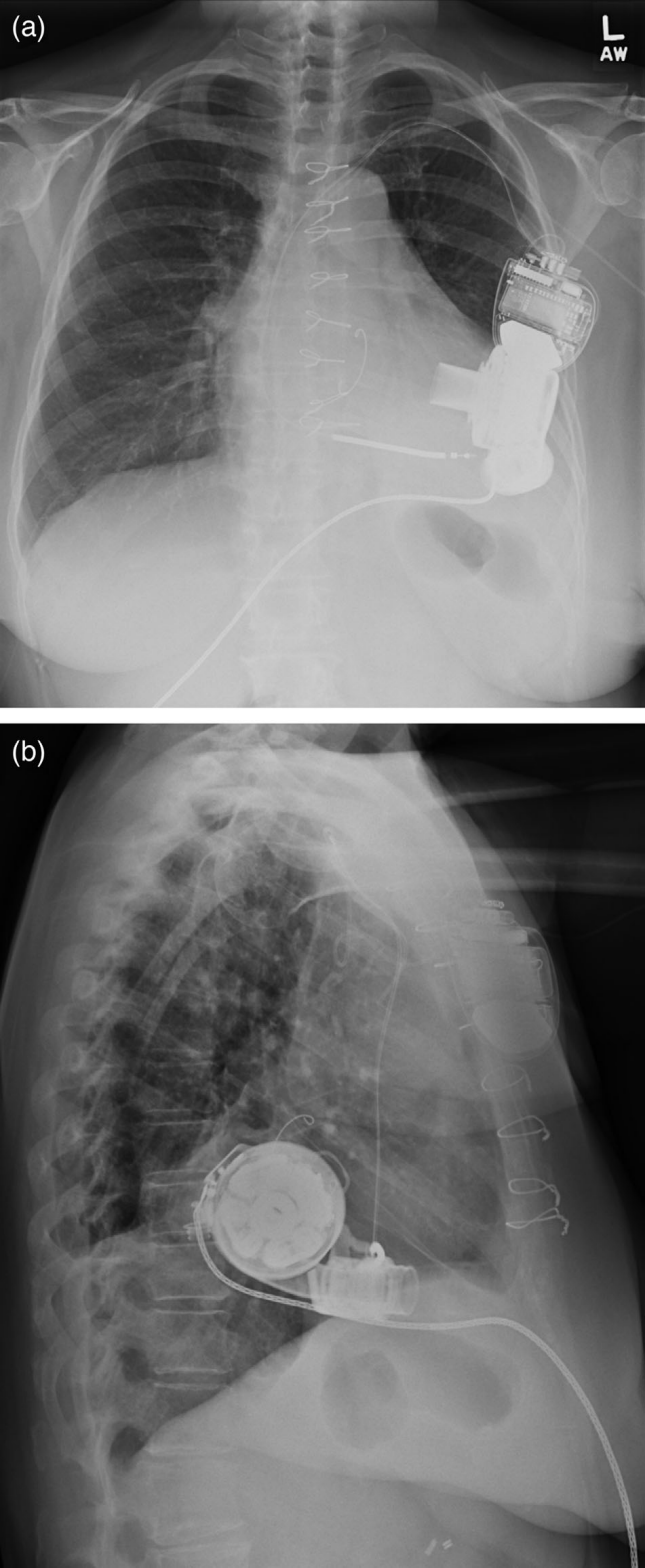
Chest radiograph exam demonstrating the relative location and proximity of the patient's LVAD to the left breast. Note this exam was obtained with computed radiography (CR) technology and not digital radiography (DR). In contrast to the patient's affected mammogram exam, LVAD related artifacts are absent on this chest radiograph. This is in keeping with a prior study by Wells et al.^19^ that demonstrated LVAD induced artifacts to affect only DR chest radiograph exams and not CR. (a) Frontal view. (b) Lateral view.
